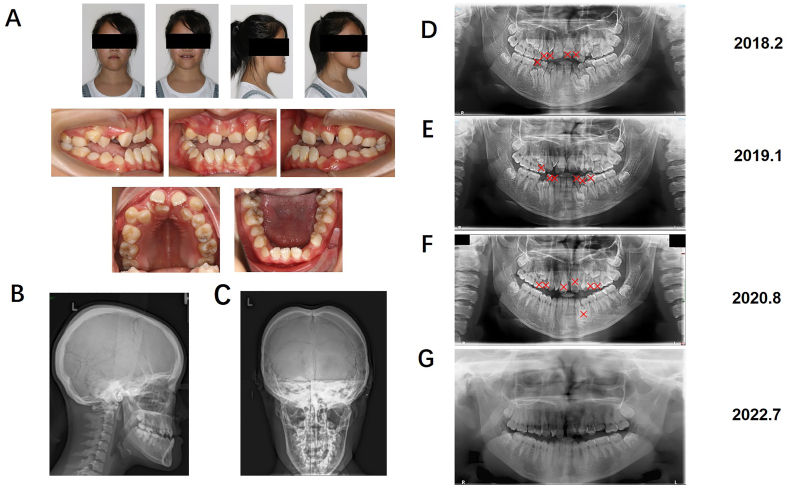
Clinical and radiographic findings of the proband. A Frontal bossing in the pretreatment facial photographs and malocclusion in the pretreatment intraoral photographs in 2019.8. B Incomplete closure of the cranial suture in the lateral cephalometric radiograph. C Skull orthograph showing incomplete closure of the fontanel. Chest radiograph demonstrates a cone-shaped thorax and hypoplasia of clavicles. (D–G) A panoramic radiograph shows the process of sequential extraction. Each red cross represents a tooth that is scheduled to be removed, including four supernumerary teeth (14 ^P^,11 ^P^,21^P^,34 ^P^) and 14 retained deciduous teeth (52–55, 61–62, 64–65, 73–75, 83–85) (not including the third molars, which had to be followed up on). (For interpretation of the references to colour in this figure legend, the reader is referred to the Web version of this article.)
